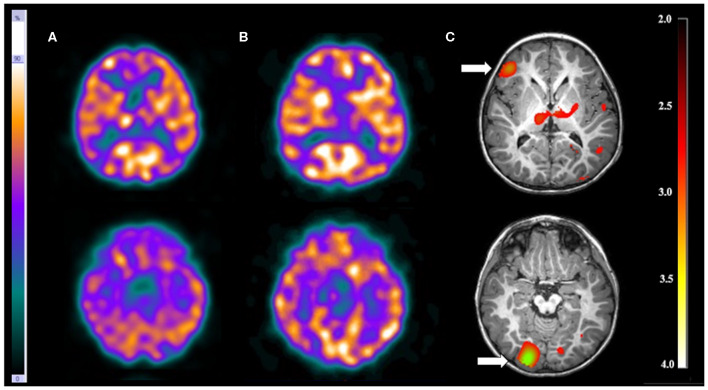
Patient #11 had tuberous sclerosis and refractory epilepsy. The axial images of the (A) ictal SPECT and the (B) interictal SPECT visual analysis showed a possible epileptogenic zone in the right frontal lobe. (C) The axial images in the SISCOM performed with SPM showed two foci of hyperperfusion in the right frontal and right occipital lobes (arrows). MRI showed the largest tubers in these two regions, and compatible with the main epileptogenic zone identified by EEGs and long-term video-EEG. This patient was submitted to surgery with resection of both tubers, resulting in cessation of seizure.
